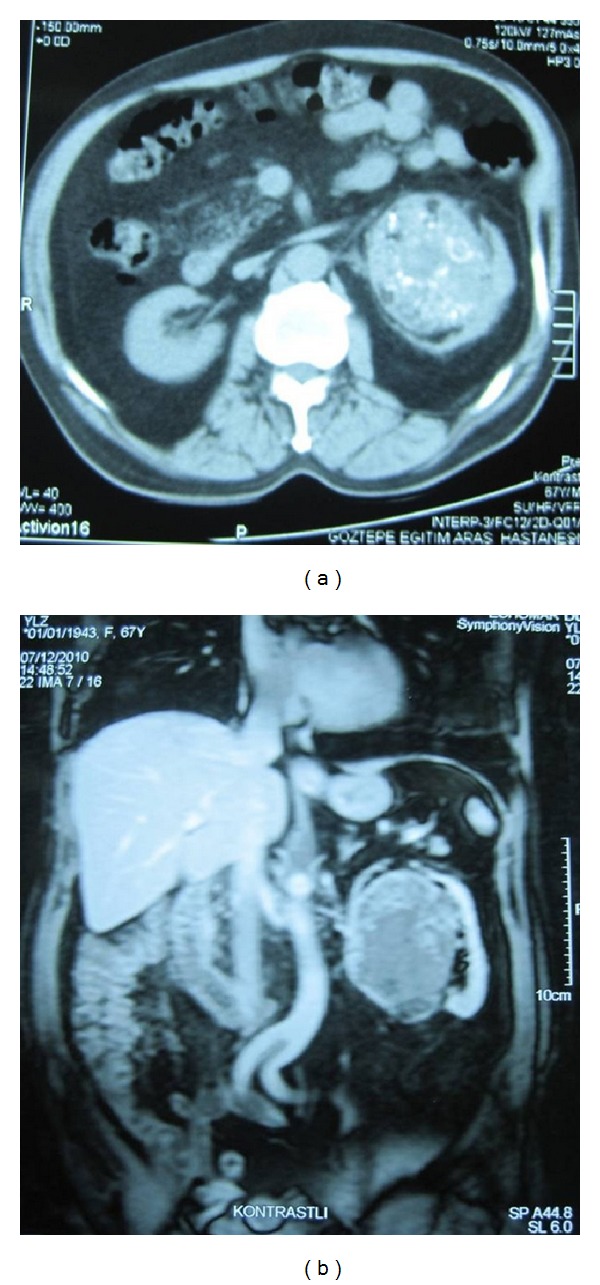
(a) Unenhanced CT scan of left kidney, showing areas of calcifications corresponding to metaplastic bone. (b) Coronal imaging of MR reveal a well-defined, solid tumor of left kidney with heterogenous enhancement.
